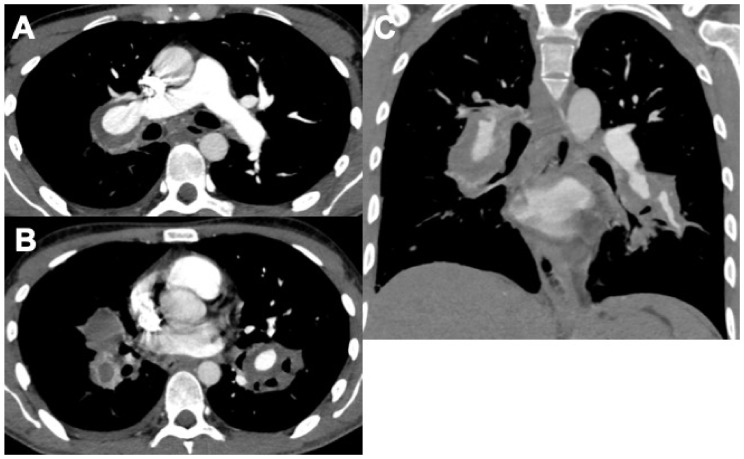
A case of pulmonary arterial involvement in Beçhet’s syndrome, showing large bilateral pulmonary artery aneurysms with eccentric thrombosis, in a young male with massive hemoptysis who arrived at the emergency department. The figures are a CT pulmonary angiography (CTPA) study: (A,B) in the axial plane and (C) in the coronal one.
